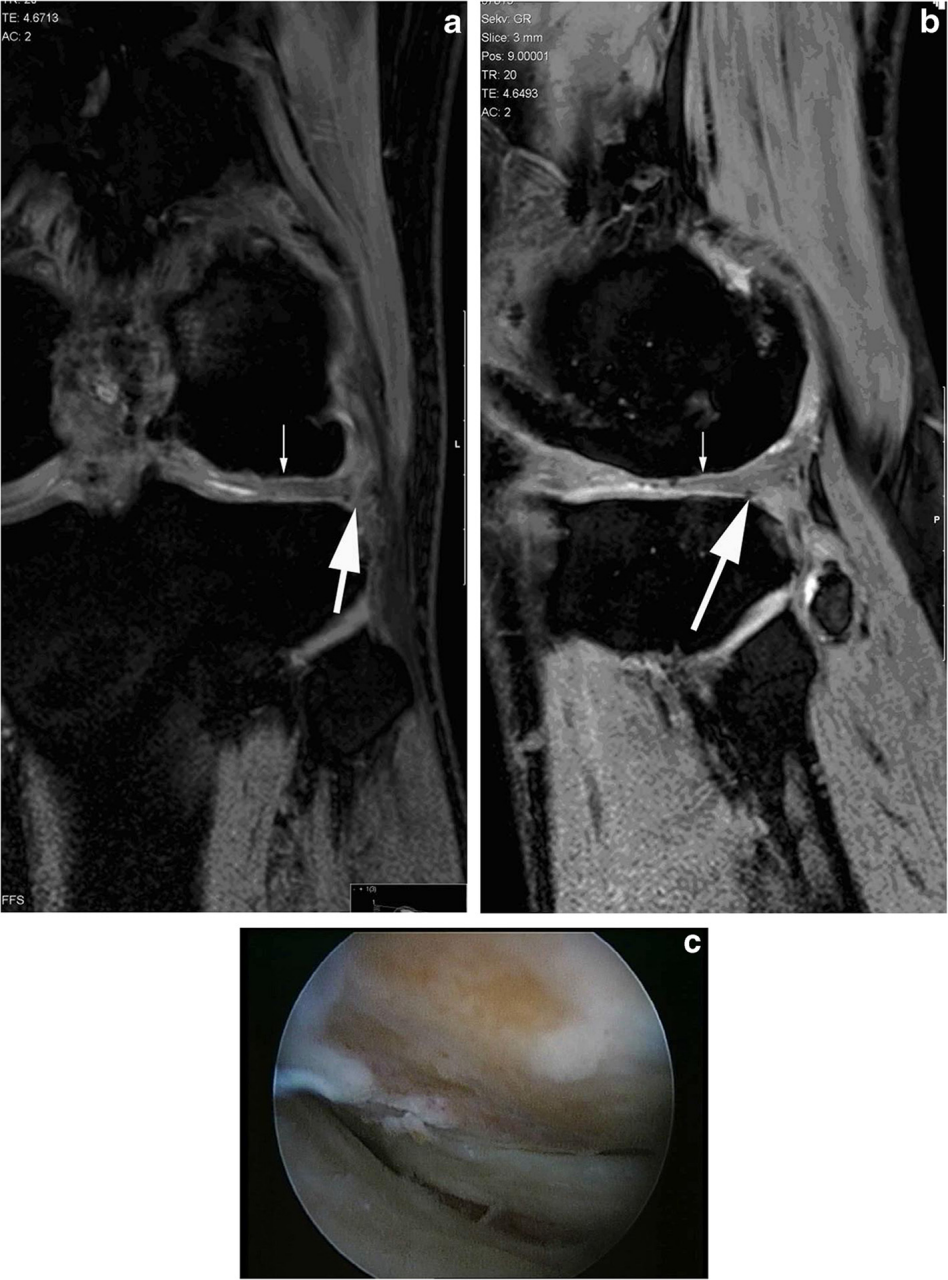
Magnetic resonance imaging of the knee on presentation. Anterior cruciate ligament reconstruction and two microfracture procedures had been performed on the left knee during the course of the last seven years. (a) Sagittal and (b) coronal three-dimensional gradient echo T1 (WATSf; repetition time 20, echo time 5.2, field of view 150, fractional anisotropy 15°, slice thickness 3 mm) left knee magnetic resonance images demonstrate a large cartilage defect on the lateral femoral condyle (arrow). Hypointense metallic artifacts due to the anterior cruciate ligament reconstruction and osteophyte formation (thick arrow) were also observed. (c) An Outerbridge grade 4, Noyes grade 3B 3 cm × 3 cm large chondral defect with a rough surface at the lateral condyle was diagnosed.
